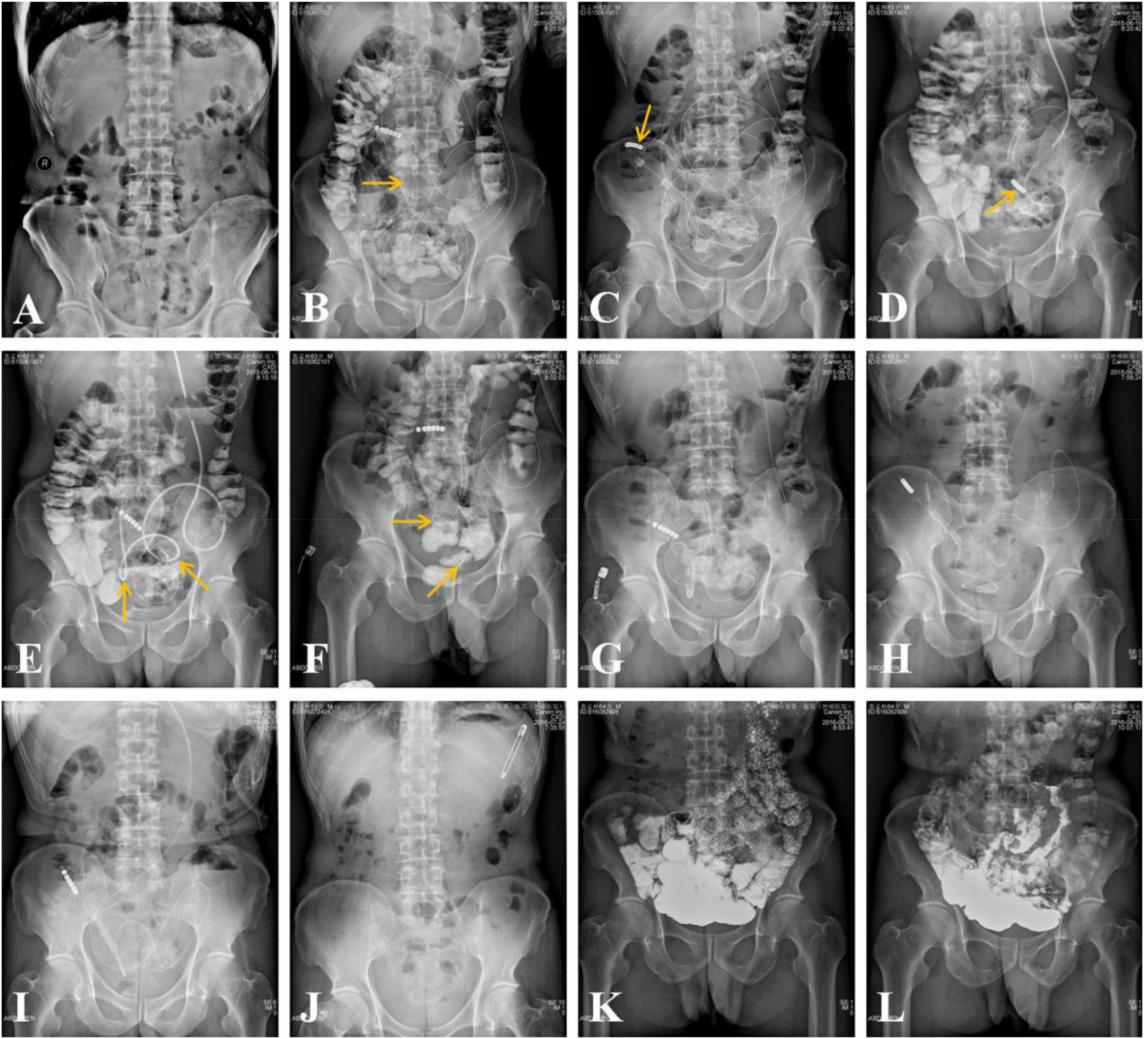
In a 63-year-old male patient, adhesive small bowel obstruction (ASBO) occurred six months following a surgical small bowel resection, which was initially performed to address ASBO secondary to a surgical repair of a stomach perforation that took place 30 years prior. (A) An erect abdominal plain radiograph reveals evidence of small bowel obstruction. (B) A transnasal ileus tube was inserted into the small bowel under fluoroscopic guidance, with the anterior balloon (indicated by an arrow) inflated with air. (C) Three days post-placement, the tip of the ileus tube (indicated by an arrow) reached the right colon. (D) The process of retracting the ileus tube to the proximal small bowel took approximately 13 min. (E) During the retraction, two obstructive sites (indicated by arrows) were identified. (F) Four days post-placement, the retraction of the ileus tube to the proximal small bowel took approximately 5 min, with the two obstructive sites (indicated by arrows) still evident, despite positional changes. (G-I) Erect abdominal plain radiographs taken 7, 10, and 15 days post-placement demonstrate a gradual loosening of the adhesive obstruction zones. The morphology of the small bowel transitioned from small to large, from numerous to sparse, and from fixed to flexible. (J) The ileus tube was removed 37 days after placement. (K,L) Gastroenterography demonstrates unobstruction of small bowel 6 months after the procedures.
